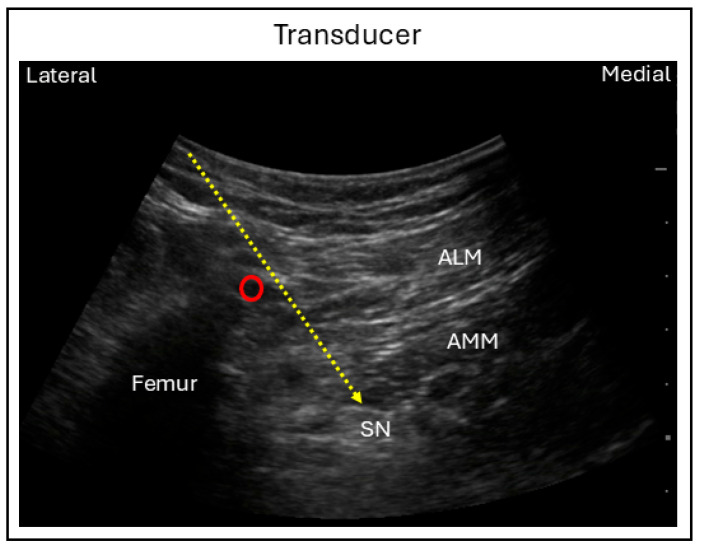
Ultrasound image demonstrating sciatic nerve block using the anterior technique. Red circle = Femoral artery; SN = Sciatic nerve; ALM = Adductor longus muscle; AMM = Adductor magnus muscle.
